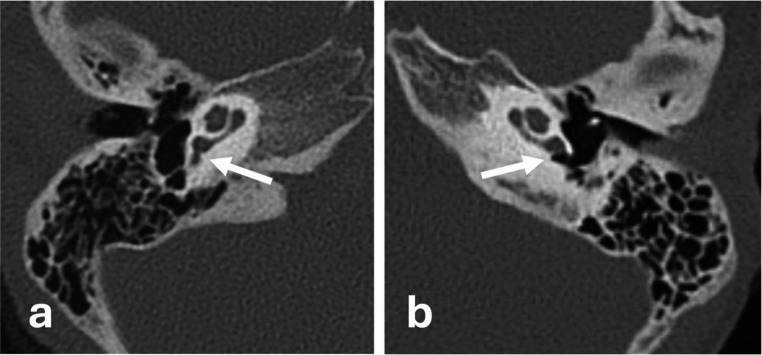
Round window hypoplasia. (a) Axial CT showing hypoplastic round window which may limit access for electrode insertion and necessitates cochleostomy approach, as opposed to (b) a normally developed round window
